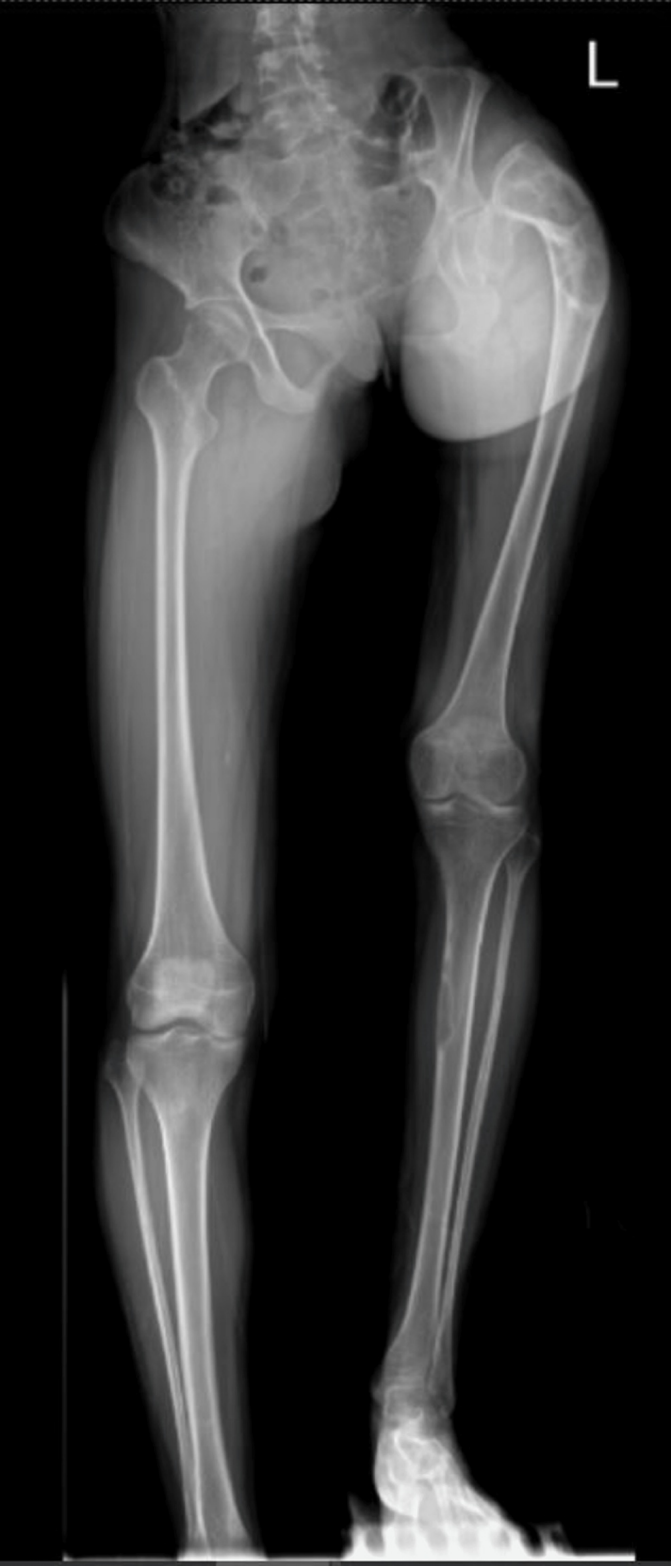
Preoperative plain radiograph of the lower limb showing severe limb length discrepancy caused by the shepherd's crook deformity of the left proximal femur.
